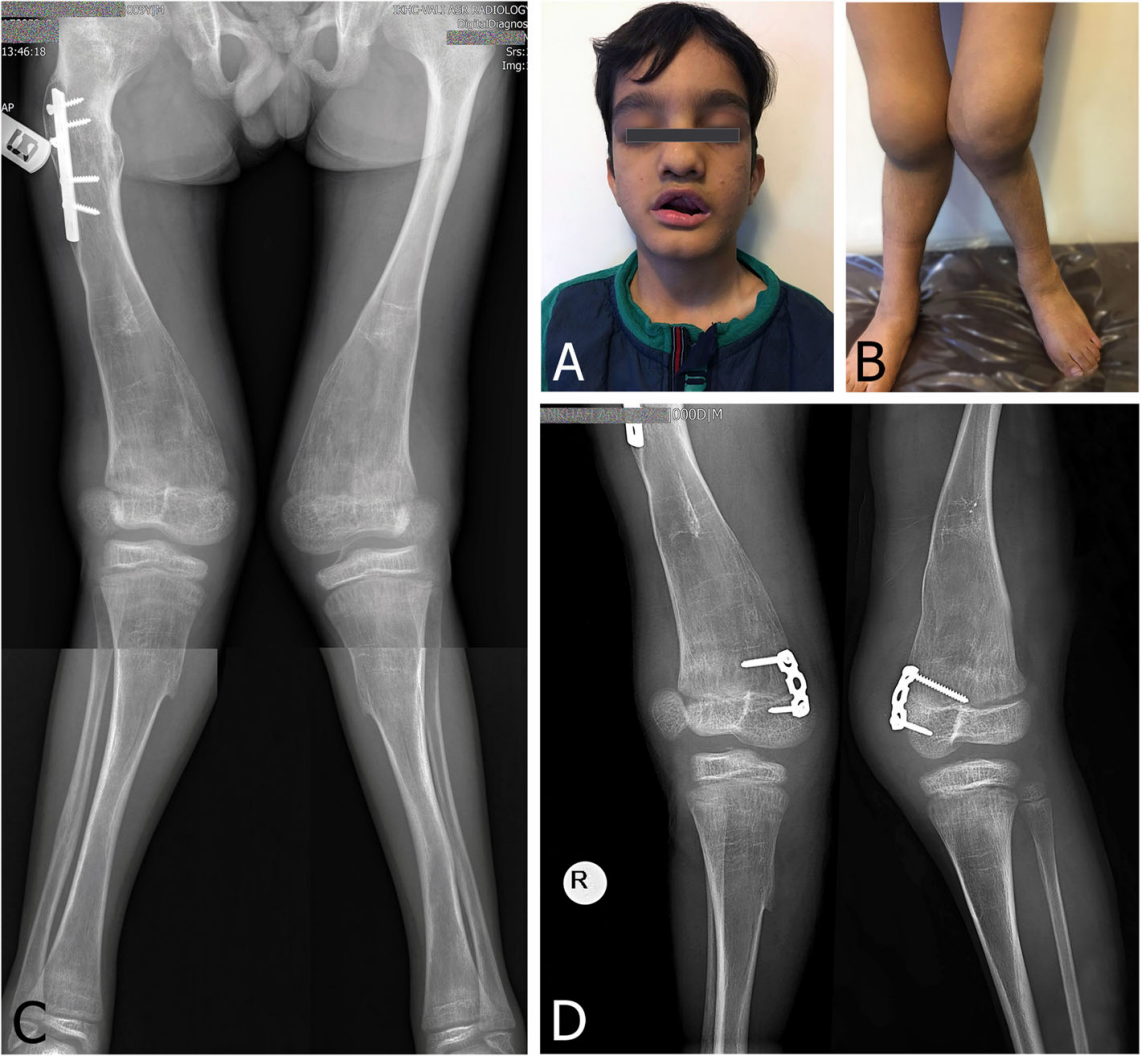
a Case 2, a 9-year-old male with HSAN type IV lip and tongue bites and loss of multiple teeth. However, this patient did not show self-mutilating behavior. b Clinical photograph at presentation, with severe bilateral swelling of the knees, and genu valga. c Standing AP radiograph, showing genu valgum. A previous femoral fracture was fixed with a plate. d Standing anteroposterior radiograph 2 years postoperative, showing improved lower limb alignment. The patellae seem dislocated on both radiographs. However, this patient has severe rotational malalignment of the lower limbs, with “apparent” patellar dislocation due to tangential X-ray projection
